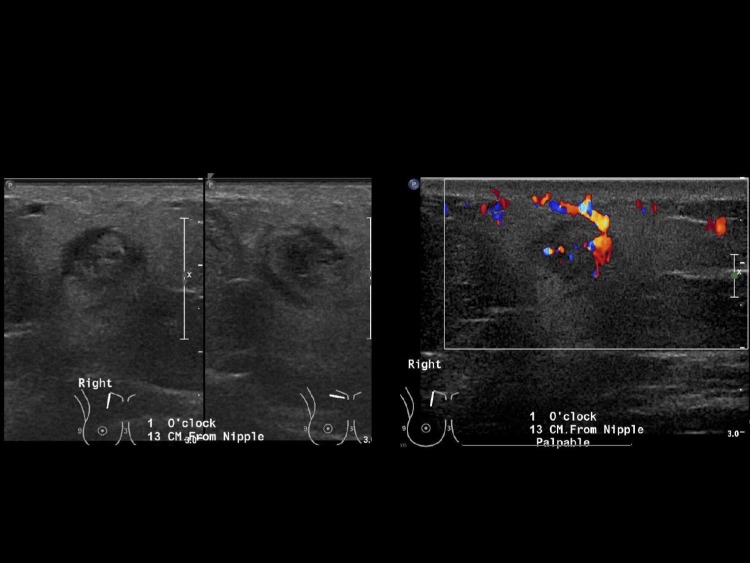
Grayscale and color ultrasound images demonstrate a solid mass with internal vascularity at 1 o’clock 13 cm from the nipple.
